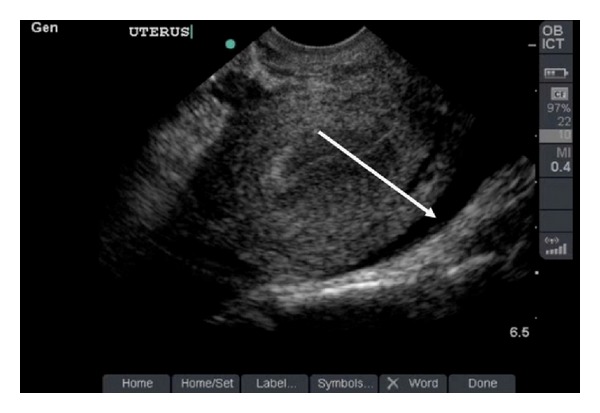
Pelvic ultrasound demonstrates the same hypoechoic fluid collection posterior to the uterine wall seen on transabdominal ultrasound in Figure 2.
